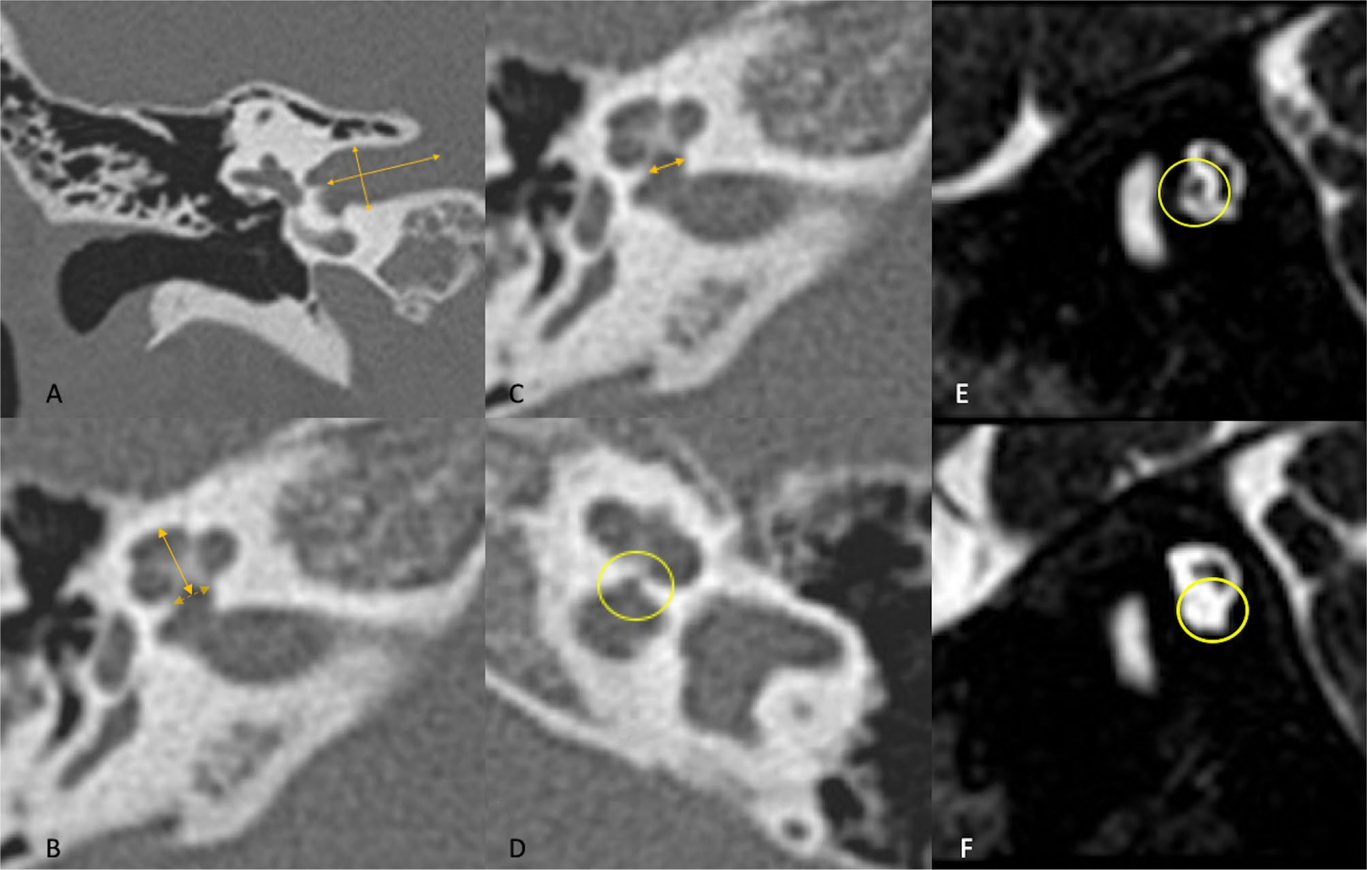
Inner ear CT scans with measures of inner auditory canal, cochlear height, cochlear aperture, and MR images of cochlear nerve. (A) Inner auditory canal (CT coronal view, right ear, normal); (B) Cochlear height (CT axial view, right ear, normal); (C) Cochlear aperture (CT axial view, right ear, normal); (D) Severe cochlear aperture stenosis (CT axial view, left ear); (E) Normal cochlear nerve, the bony canal is well visible and comparable with the facial nerve bony canal; (MR oblique-sagittal view); (F) Hypoplastic cochlear nerve, the bony canal is almost completely ossified, (MR oblique-sagittal view).
